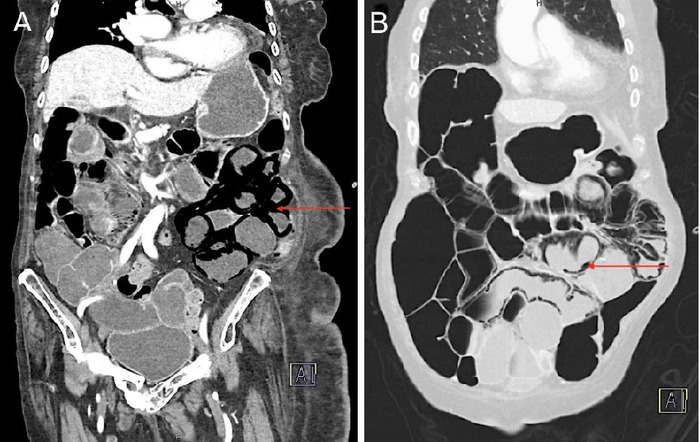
Computed tomography with intravenous contrast (A, B) coronal views of the abdomen and pelvis show diffuse pneumatosis intestinalis (arrows) in the small bowel.
